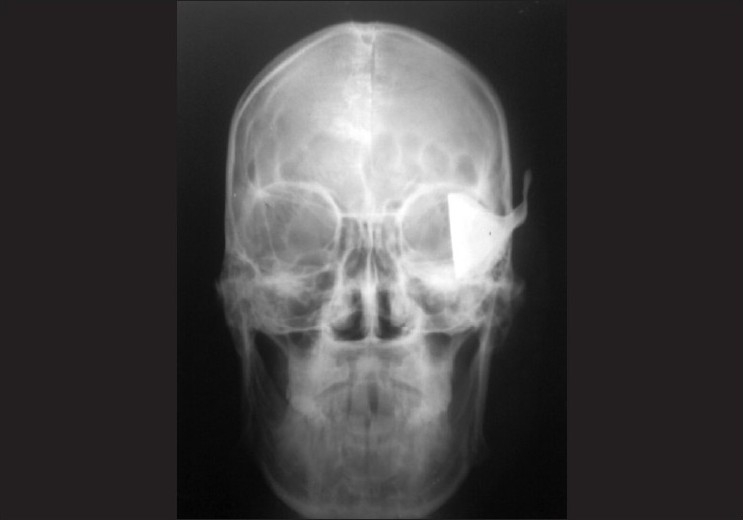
X-ray film showing handle of spoon in soft tissue with intact bony anatomy
